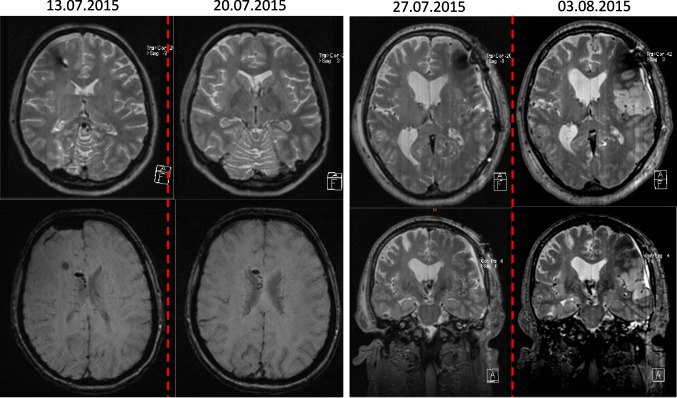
On the left: acute and subacute MRI showing little to no difference in lesion load. On the right: acute and subacute MRI showing clear dynamic on lesion load with hypointensity in the left frontotemporal region
